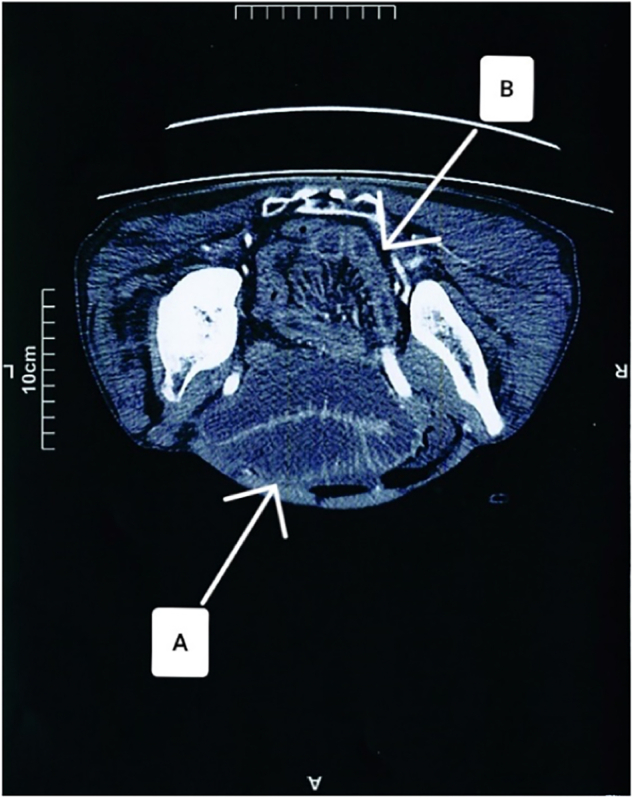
CT scan of the patient's abdomen; Flash A indicates dilated bowel loops, Flash B indicates normal bowel loops.
